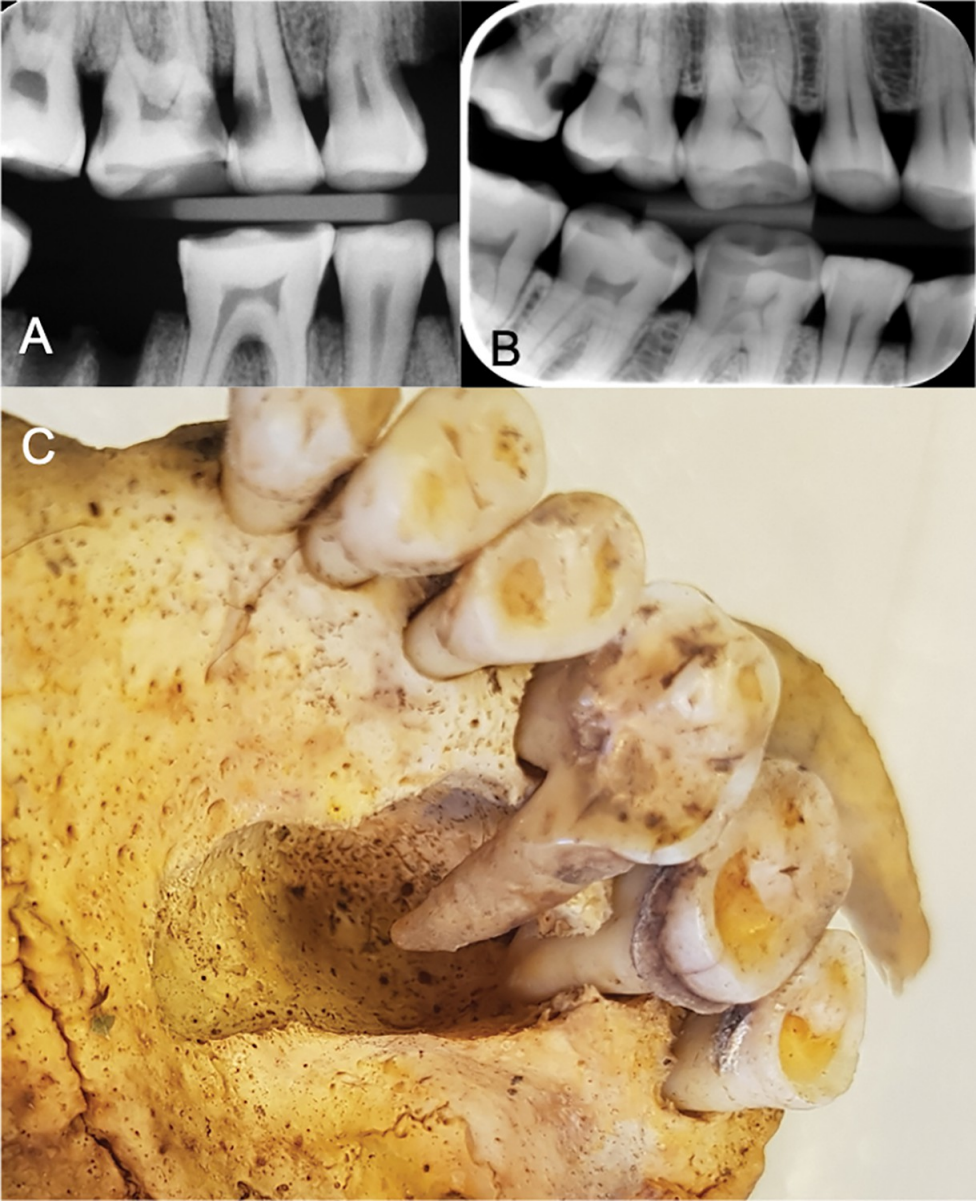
Dental pathological conditions. A) Manifest carious lesions in the first maxillary molar and second maxillary bicuspid, B) Manifest carious lesions in the second and third maxillary molars and C) Periapical lesion on molar 26 spreading medially in the palate. Possibly a cyst.
